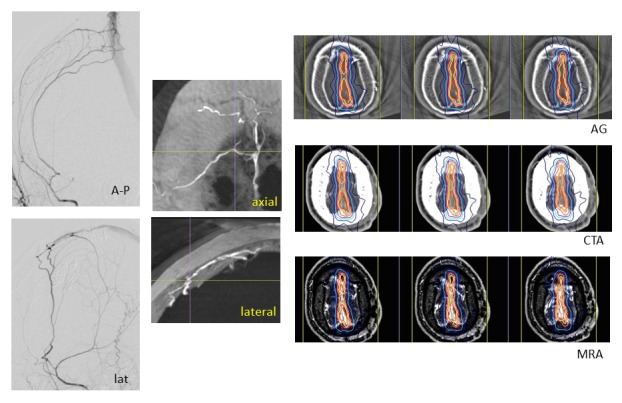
A dural arteriovenous fistula (AVF). Digital subtraction angiography showing a dural AVF of the superior sagittal sinus (left). The 3-D reconstruction from axial images of rotation angiography (middle line) showing fistulas to the sinus. The fistula points are clearly shown in 3-D images (crossed line). The dose plan targeting the AVF and the sinus wall on axial images of an angiogram (AG) fused to those of CT angiogram (CTA) and enhanced MR angiogram (MRA).
